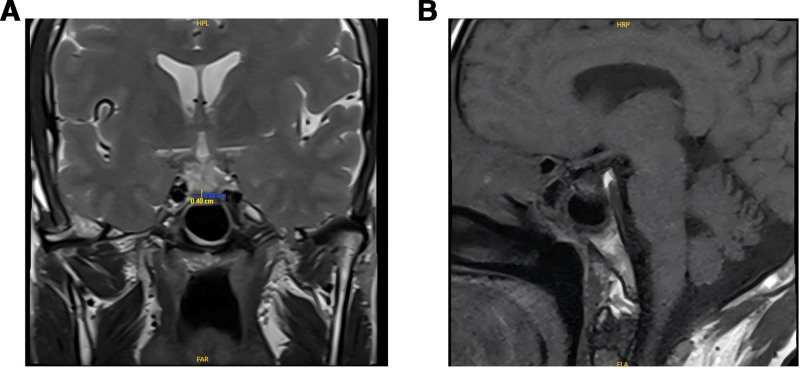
Pituitary MRI: (A) coronal; (B) sagittal. MRI = magnetic resonance imaging.
